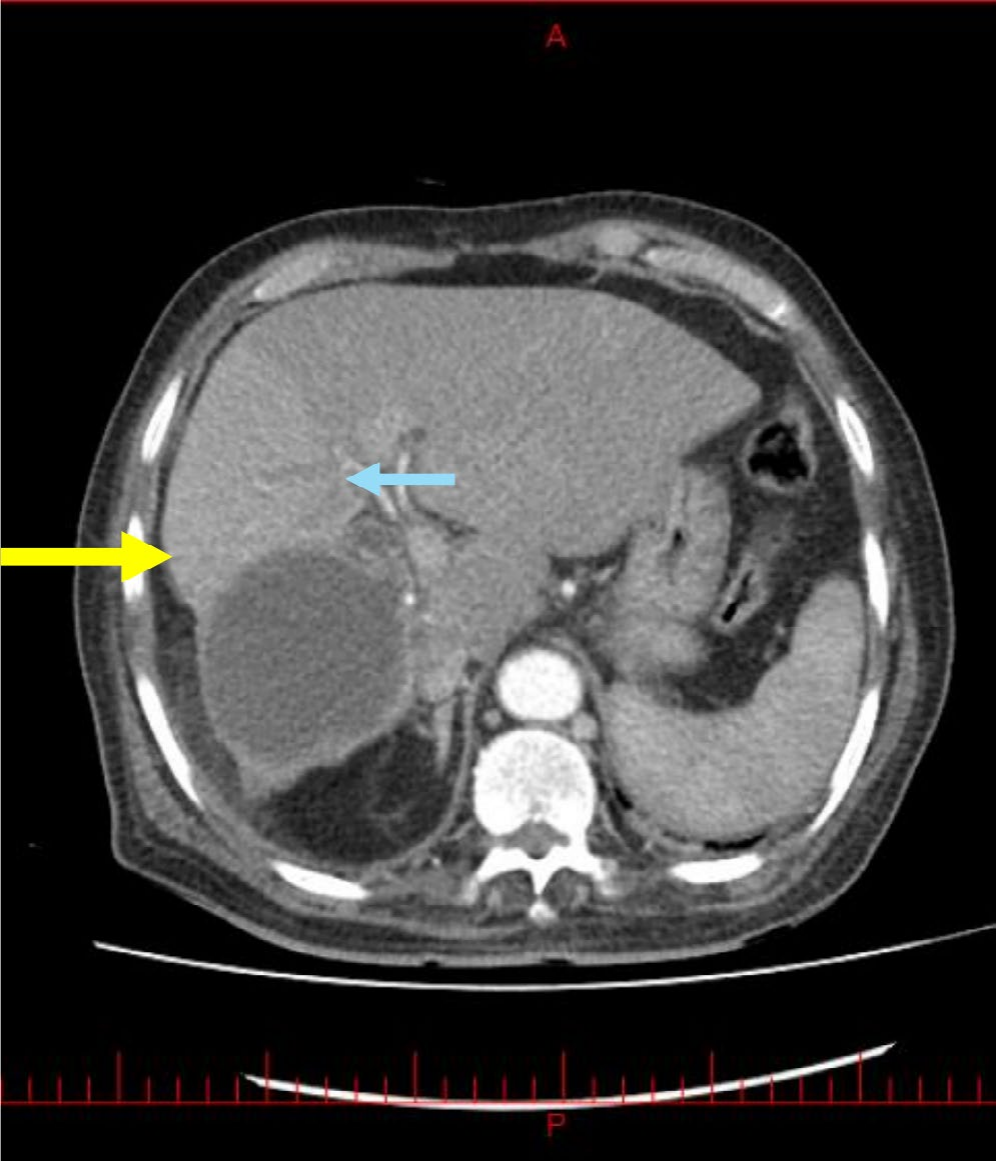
CT of the abdomen (axial view). A hydatid cyst is observed in the right lobe of the liver (yellow arrow), alongside a fistula connecting the cyst to a branch of central biliary tree (blue arrow), which leads to the CBD (green arrow).
